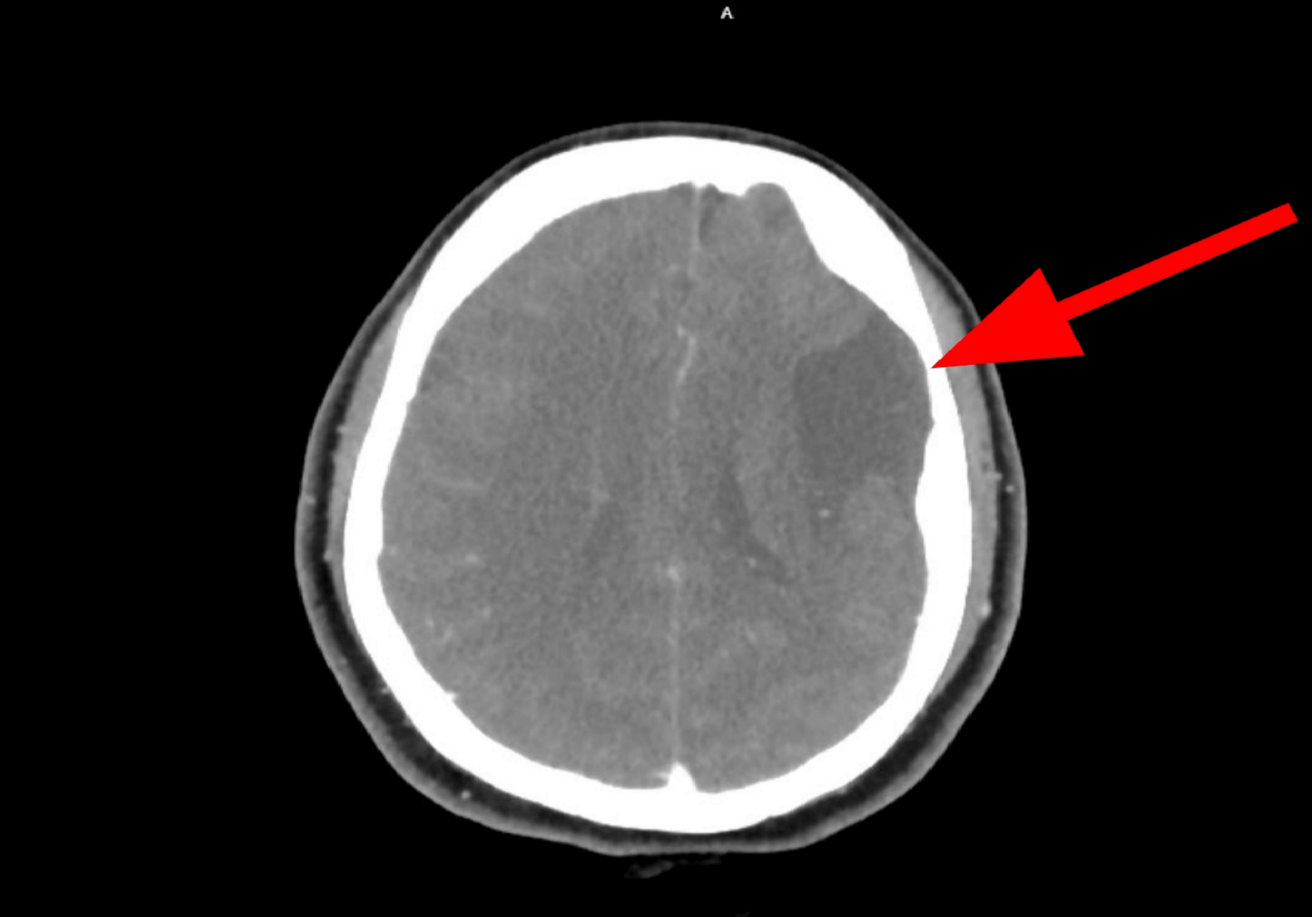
CT image showing a possible previous infarct in the left frontal lobe (red arrow) with no other abnormalities detected CT: computed tomography
